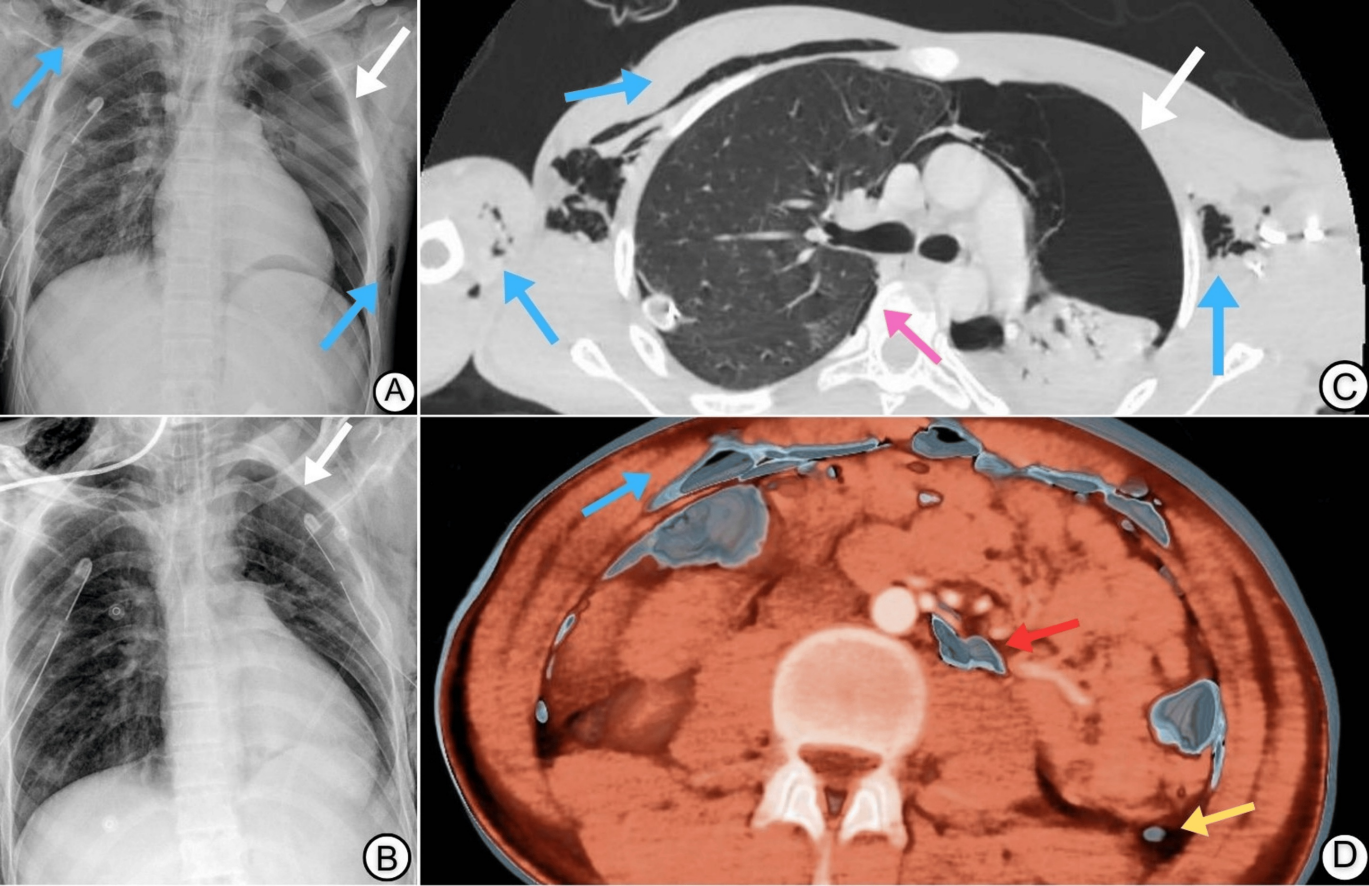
Chest and abdominal imaging findings in a trauma patient with the Macklin effect. The initial X-ray (A) showed a large left-sided pneumothorax (white arrows) with air accumulating in the pleural space, and subcutaneous emphysema (blue arrows). The follow-up X-ray (B) revealed the placement of a chest tube (white arrow), with partial re-expansion of the lung and some residual subcutaneous emphysema. (C) An axial computed tomography (CT) scan of the chest highlighted a significant left-sided pneumothorax (white arrow) with extensive air in the mediastinum (pink arrow) and subcutaneous tissues (blue arrows). (D) 3D reconstruction of the abdomen CT scan showed free air in the peritoneal cavity (red arrows) and retroperitoneal space (yellow arrow), indicating pneumoperitoneum and pneumoretroperitoneum, respectively, with air tracking from the thorax into the abdomen and previously noted subcutaneous emphysema (blue arrow).
